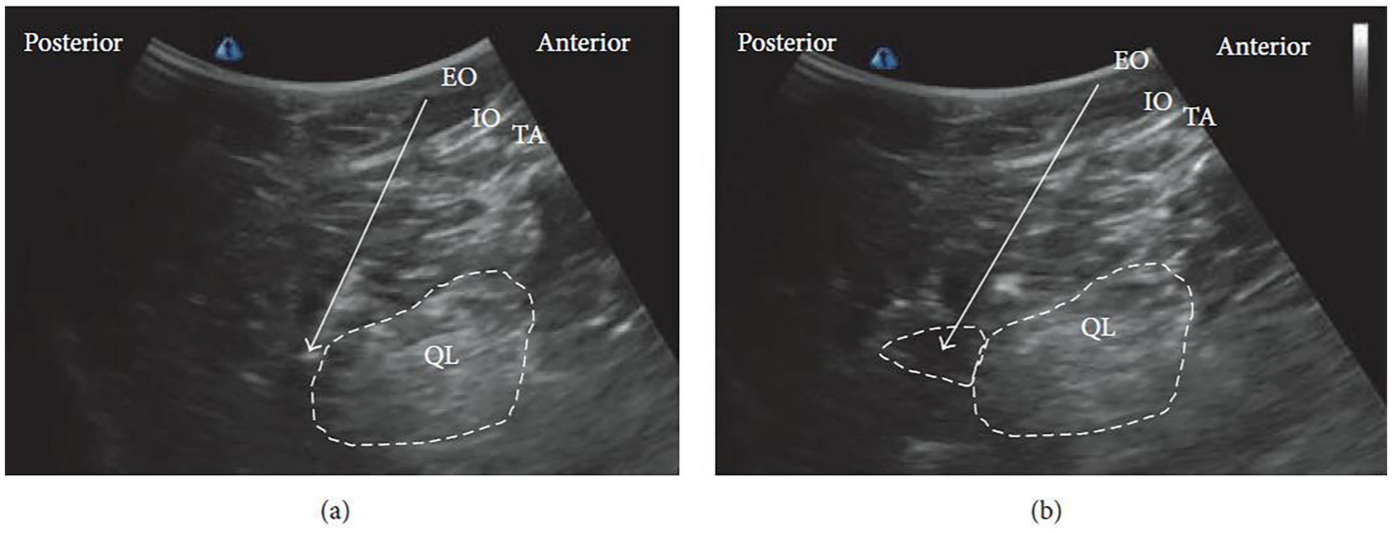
Ultrasound images of posterior QLB: (a) before injection and (b) after injection. EO, external oblique; IO, internal oblique; TA, transversus abdominis; QL, quadratus lumborum. White arrow: needle trajectory; white dotted line: spread of local anesthetic [Reproduced from Ueshima et al. (28). © John Wiley & Sons, Inc. Distributed under the Creative Commons Attribution License, CC BY 4.0].
